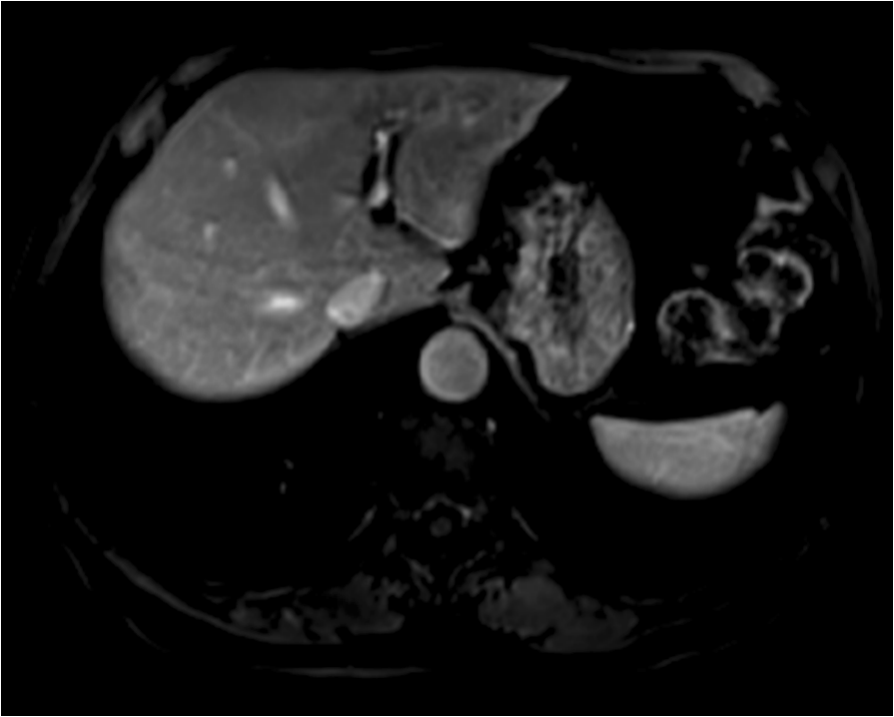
Axial three-dimensional late phase gradient echo (GRE) T1-weighted images, obtained 90 seconds after administration of intravenous contrast, demonstrating perilesional enhancement of the mass.
